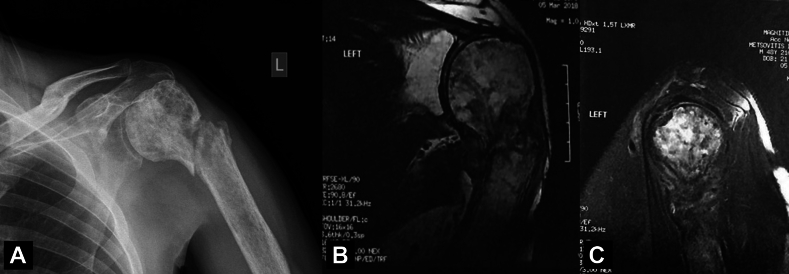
(A) Plain X-ray showing a pathological fracture of the humeral head with diffuse permeative sclerotic areas and signs of osteonecrosis, (B, C) the same findings are seen in the sagittal and coronal MRI. *MRI*, magnetic resonance imaging.
